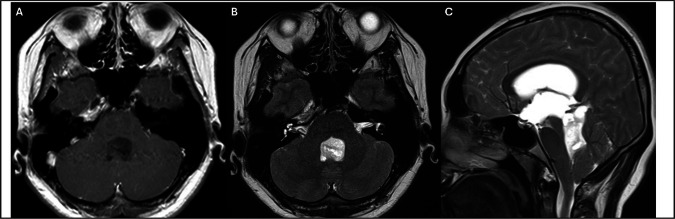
Contrast-enhanced axial T1-weighted (A) and T2-weighted (B) magnetic resonance images demonstrating a rosette-forming glioneuronal tumor prior to surgery. The lesion appears hypointense on T1-weighted images and hyperintense on T2-weighted sequences. It is primarily located in the fourth ventricle with periaqueductal extension (C). Mixed solid and cystic components are also identified
